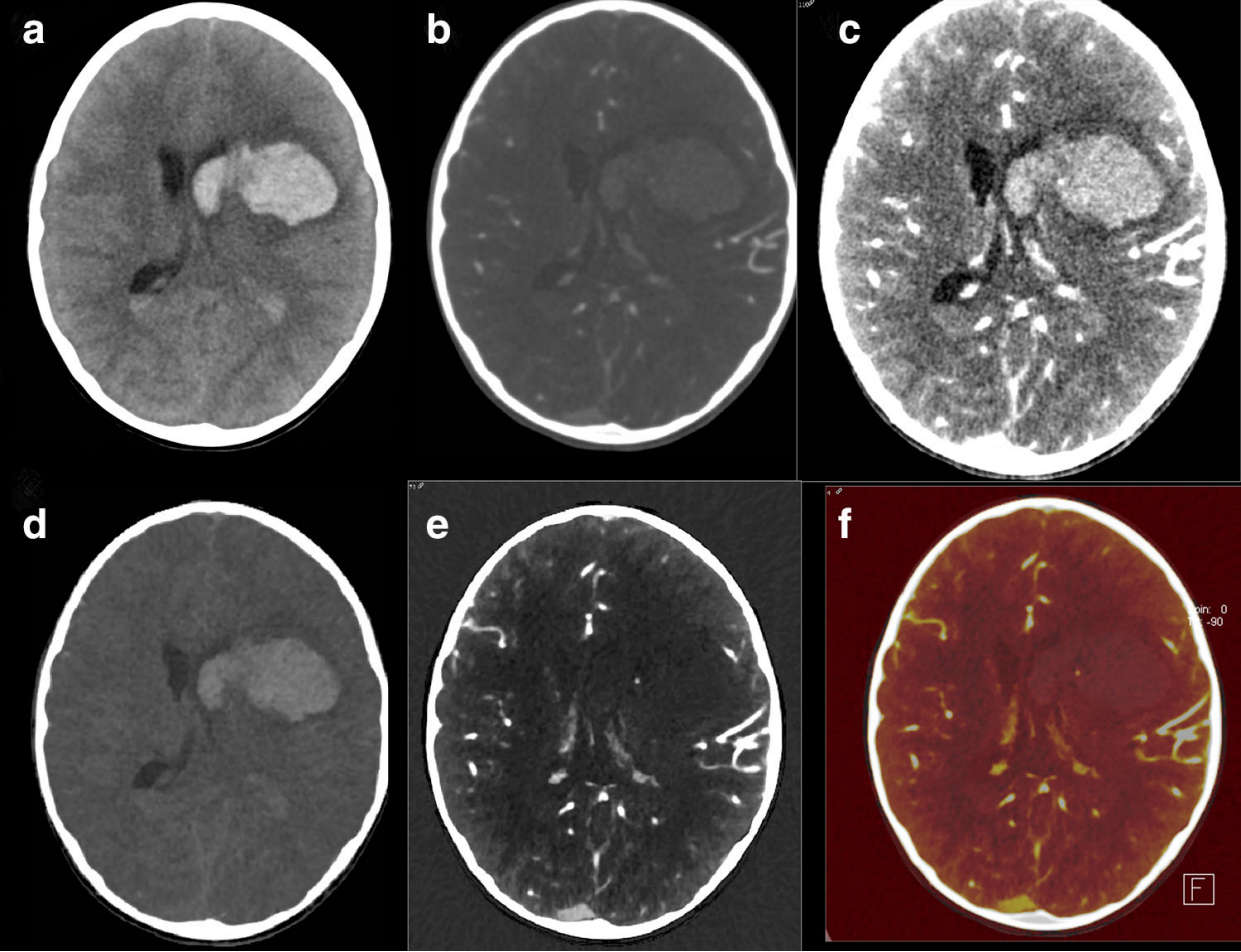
10-year-old female presented with right-sided hemiparesis based on ICH within the left hemisphere with intraventricular extension shown at NECT (*a*). A DECTA was performed. Shown are mixed images at H20 (*b*) and H31 kernel (*c*), without underlying pathology and initially read as without spot sign. VNC (*d*) visualized the hematoma. IOM (*e*) and fusion images (*f*) clearly demonstrated a spotsign centrally in the hematoma (conventional CT 120 kVp, 300 mAs, CTDI 44.47 mGy, DLP 747 mGycm. DECTA 80/Sn140 kVp, 310/155 mAs, CTDI 26.34 mGy, DLP 433 mGycm). 50 cc iodinated contrast 300 mg/ml; injection rate 2.8 cc/s; 20 cc saline flush 2.8 cc/s)
